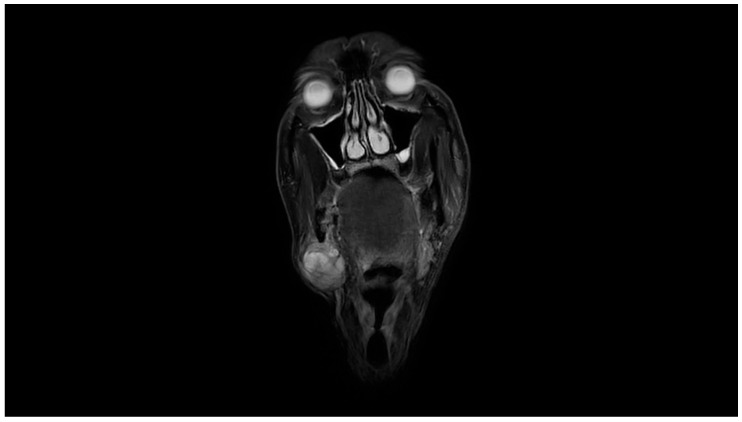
Axial contrast-enhanced magnetic resonance image of the neck demonstrating heterogeneous enhancement of the right submandibular lesion, with internal non-enhancing areas suggestive of necrotic change.
